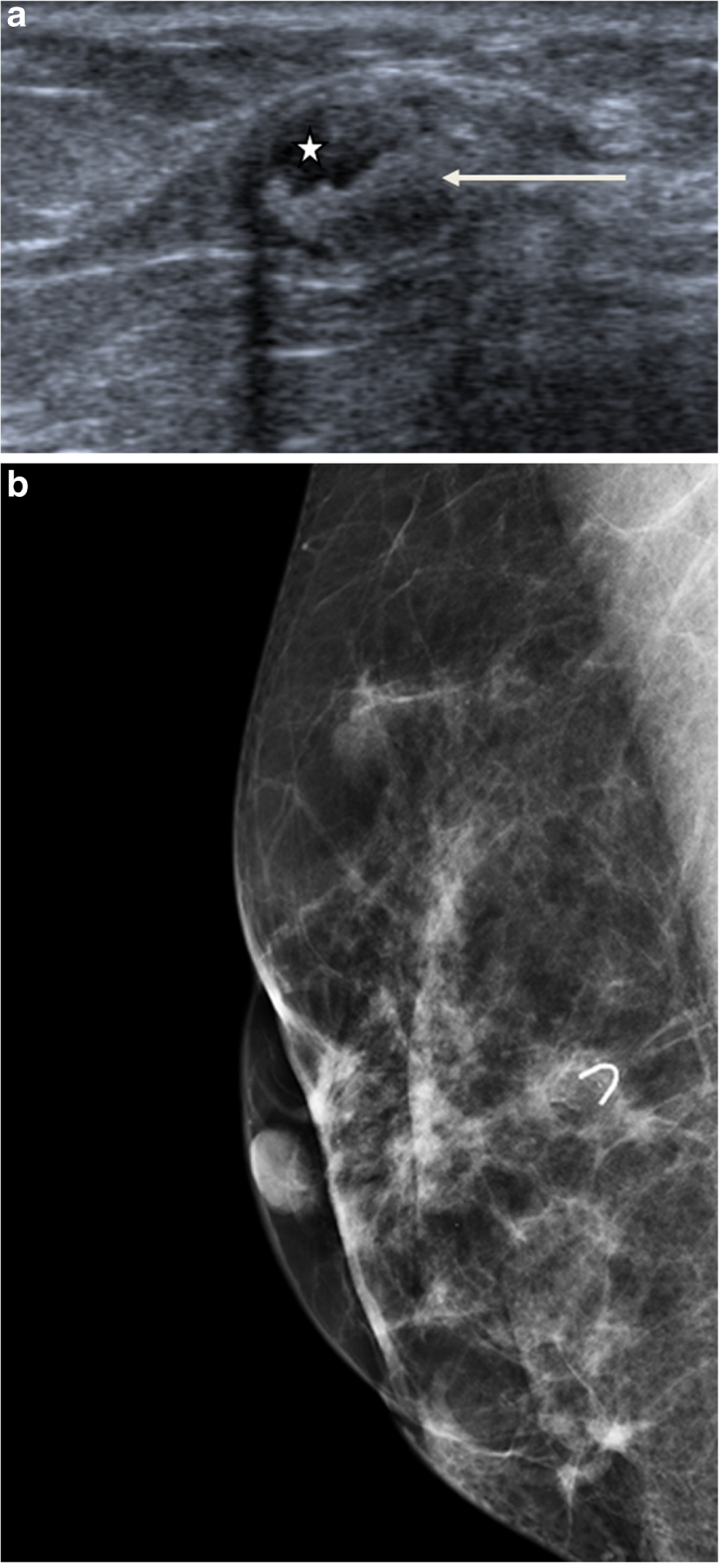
Fat necrosis. a Ultrasound scan showing a complex mass composed of an echo-free fat component (*asterisk*) and an echogenic fibrous component (*arrow*). b Mammography, oblique view, showing a radiolucent formation projected over a clip
